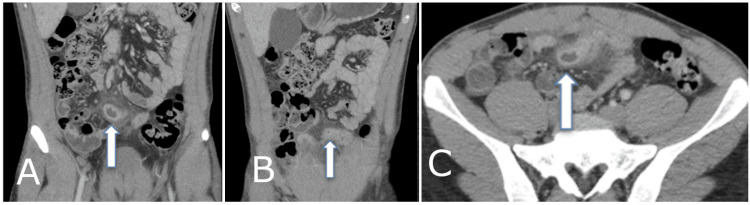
Coronal (A, B) and axial (C) views of computed tomography (CT) scan showing a blind-ending, inflamed tubular structure originating from the ileum (arrows).
